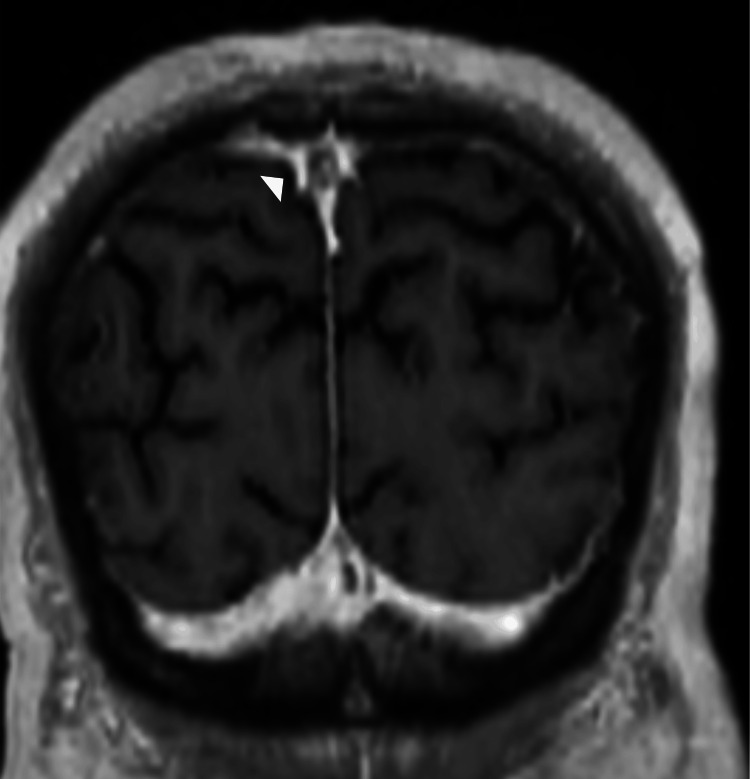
Three-month follow-up MRI coronal volumetric T1 postcontrast sequence demonstrates near complete resolution with minimal persistent thrombus in the superior sagittal sinus
